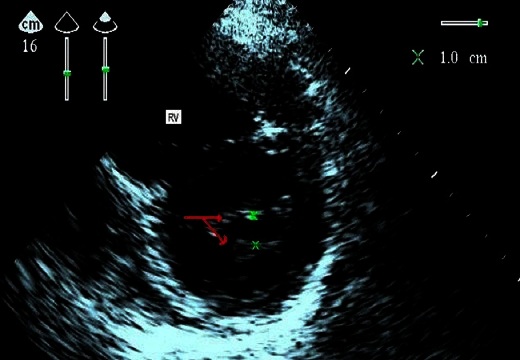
Parasternal short-axis 2D echocardiographic image in diastole showing thickening and stenosis of the mitral valve. (RV: right ventricle, arrows: thickening of the large and small mitral valve)
